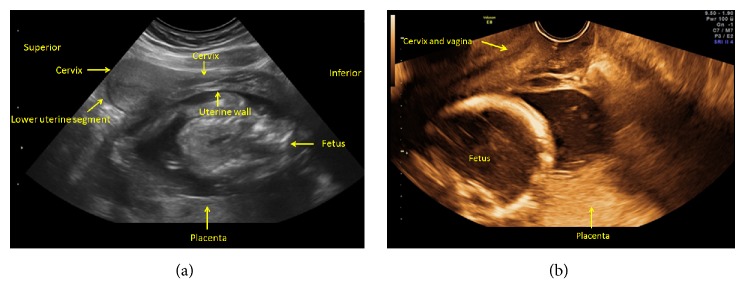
Re-evaluation of the ultrasound images obtained at 19 weeks. Transabdominal (a) and endovaginal (b) ultrasound images showing the anteriorly located and stretched cervix and lower uterine segment.
